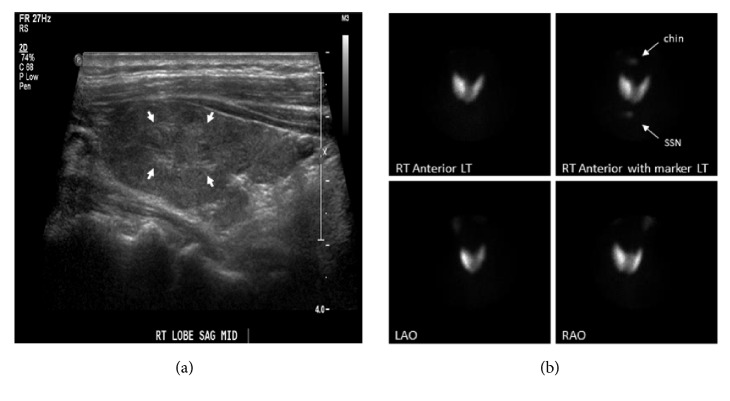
Thyroid ultrasound and nuclear scan. Grayscale sonographic image of the right thyroid lobe demonstrates a heterogeneous nodule with ill-defined borders (arrows) (a); technetium-99m pertechnetate thyroid scan showing increased uptake in both thyroid lobes with a more focal hot nodule in the superior to mid portion of the right lobe, corresponding to the nodule seen on ultrasound (b).
